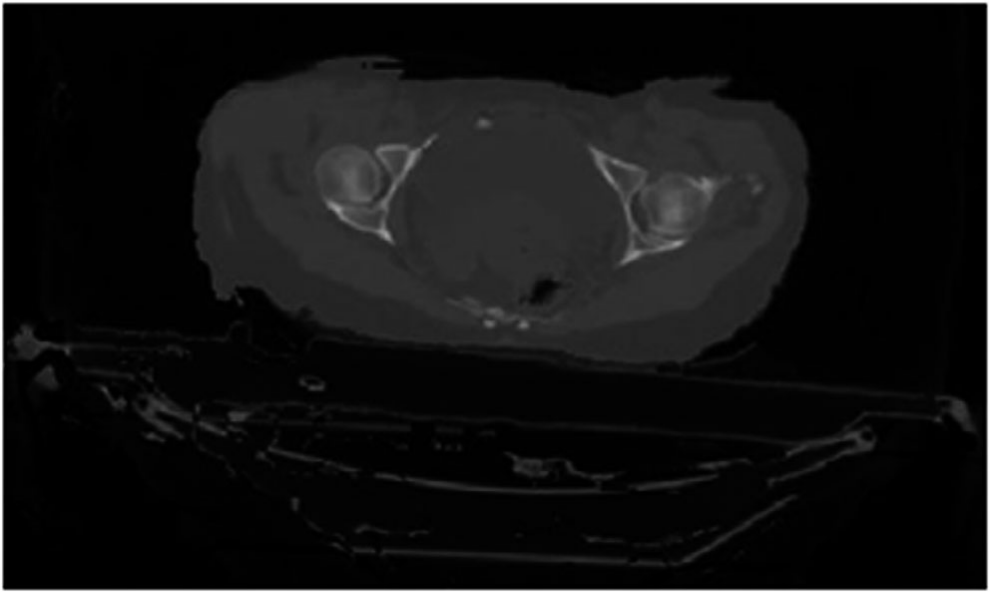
Example of error in CT deformable registration.
